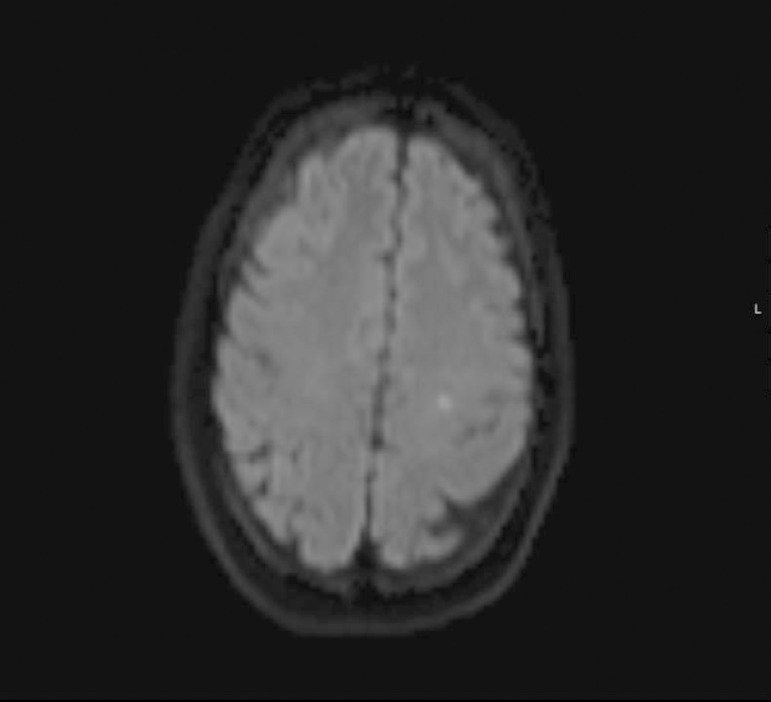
Magnetic resonance imaging (axial diffusion weighted imaging) showing a small lesion with restricted diffusion on the left corona radiata.
